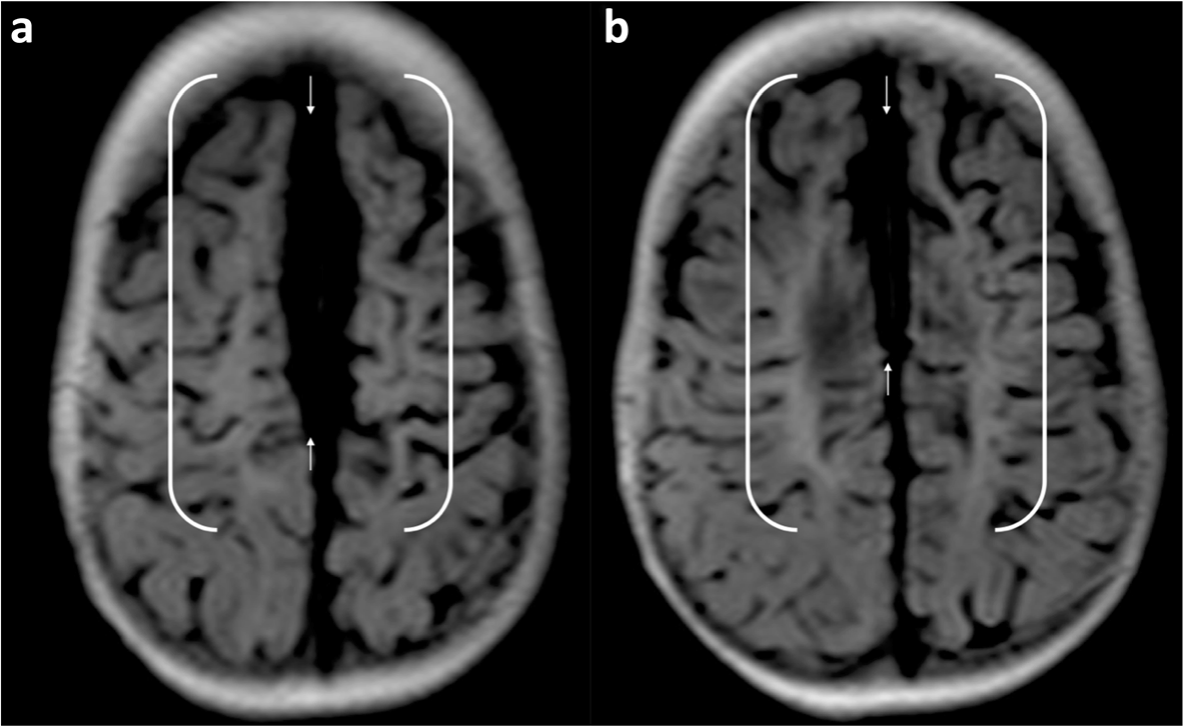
a, b Sequential axial FLAIR images in a 2-year-old girl who sustained a partial-prolonged HII at term gestation, demonstrating volume loss and abnormal signal involving the parasagittal and anterior inter-vascular watershed regions in continuity (between white brackets) and sparing of the peri-Sylvian and posterior watershed. There is bi-convex expansion of the inter-hemispheric fissure at the anterior vertex (white arrows) indicating the predominant parasagittal involvement
